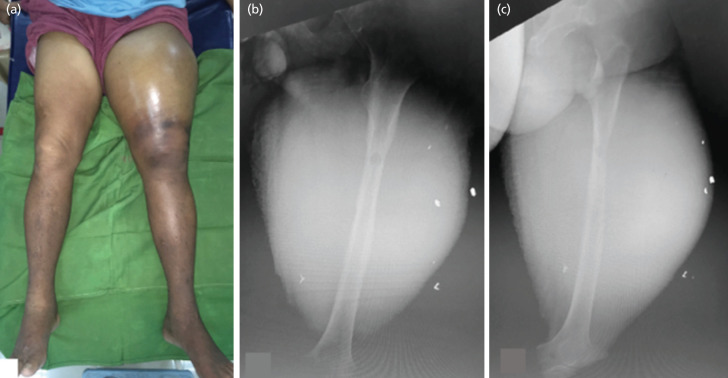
(a) Gross appearance of the bilateral lower extremities. Radiographs of the left femur in (b) anteroposterior and (c) lateral views, show a permeative lytic lesion and cortical erosion in the left femur, surrounded by a large soft tissue mass.
